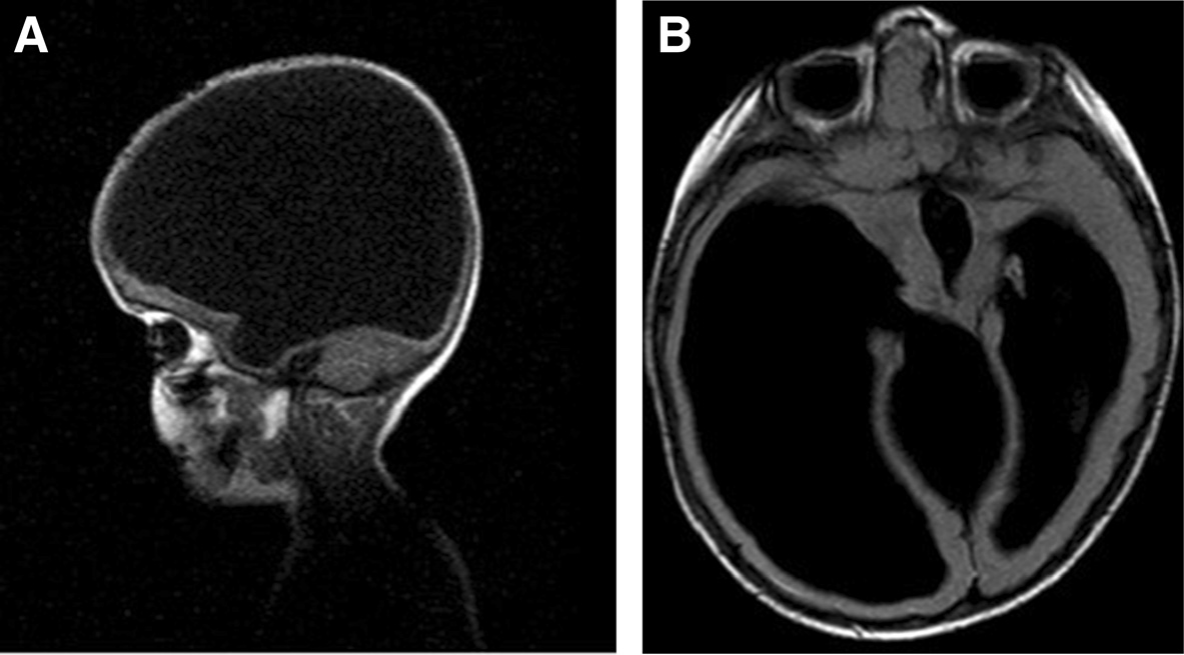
AB: Sagittal (A) and axial (B) projections of Cerebral T1-weighted MRI performed on a patient affected by severe congenital hydrocephalus. The images show the absence of cerebral hemisphere with only a small portion of the right frontal lobe and a rime of the left frontal and parietal lobes. Third ventricle is present and enlarged.
